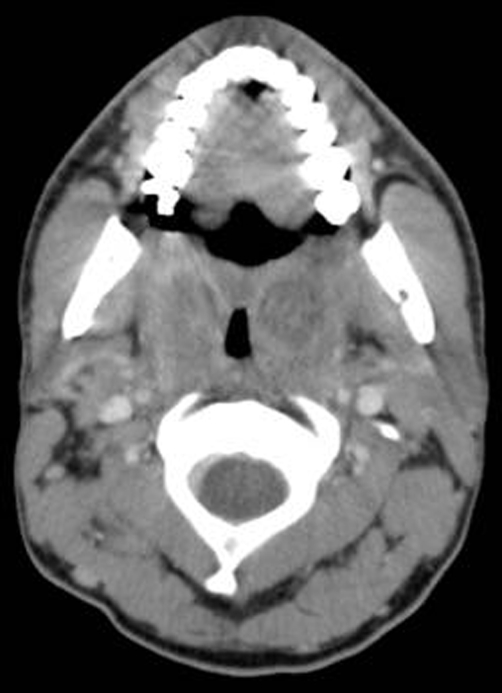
CT scan of head and neck. Note tonsillar swelling.
